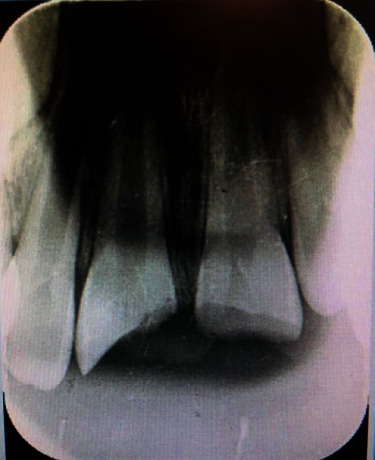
Radiographic examinations.
